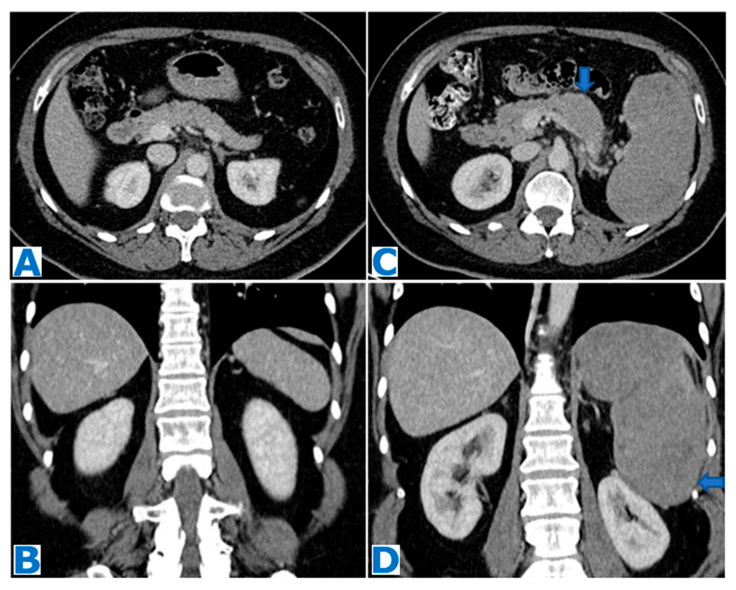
Images from 2017, (A,B): axial and coronal CE portal venous phase CT MPR. Images from 2022, (C,D): axial and coronal CE portal venous phase CT MPR. Secondary pancreatic lymphoma in a 60-year-old patient with a known glomus jugulotympanicum paraganglioma on surveillance. Note the normal appearance of pancreas and the spleen (A,B) on the prior CT. Abdominal adenopathies were found (not shown). Observe the spleen enlargement (arrow in (D)), with focal lesions. Hepatoduodenal and retroperitoneal adenopathies were also found (not shown). Note the isoenhancing mass within the body of the pancreas (arrow in (C)). EUS-guided FNB revealed a diffuse large B-cell SPL.
